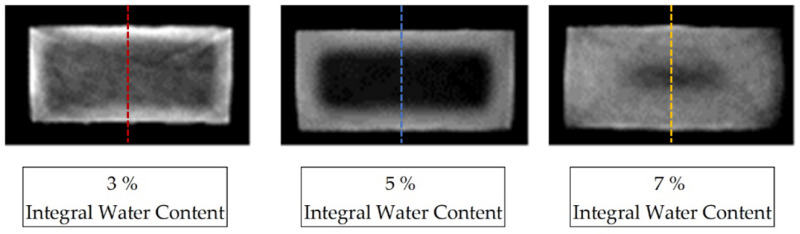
CT reconstruction of three PA6 samples with concentration distributions at different integral water contents, saturated at 23 °C in a water bath. The images have been magnified and are contrast-spread.
